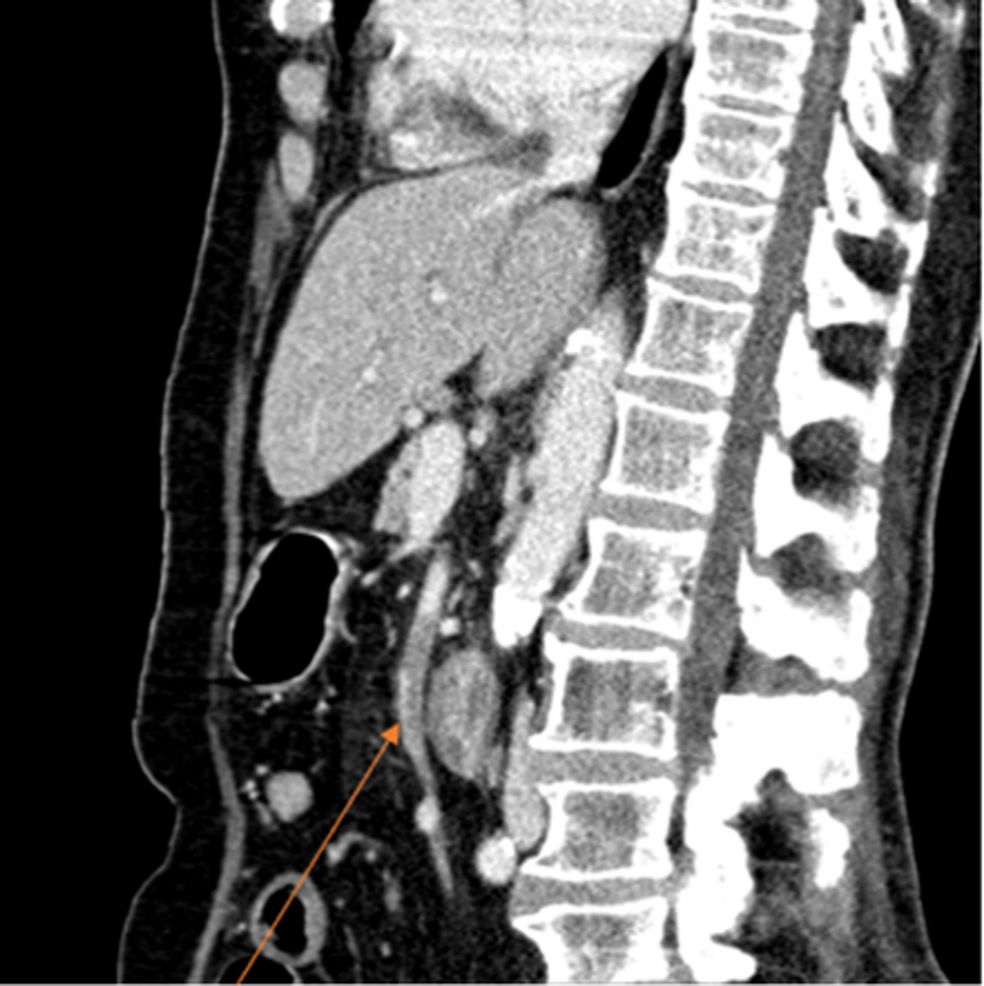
Abdominal and pelvic CT showing subtraction of the lumen of the upper mesenteric artery (arrow) CT, computed tomography
